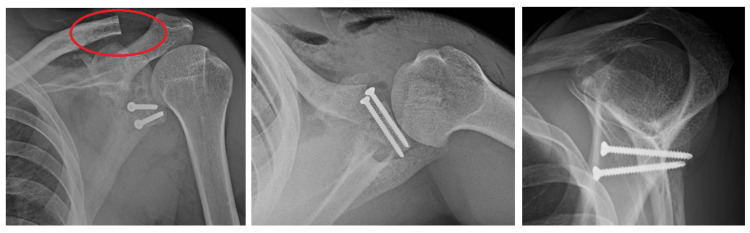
Repeat X-ray after glenoid reconstruction The red circle noted is where there is a distal clavicular resection. The rest of the figures show the internal fixation of the anterior glenoid defect with two threaded screws
